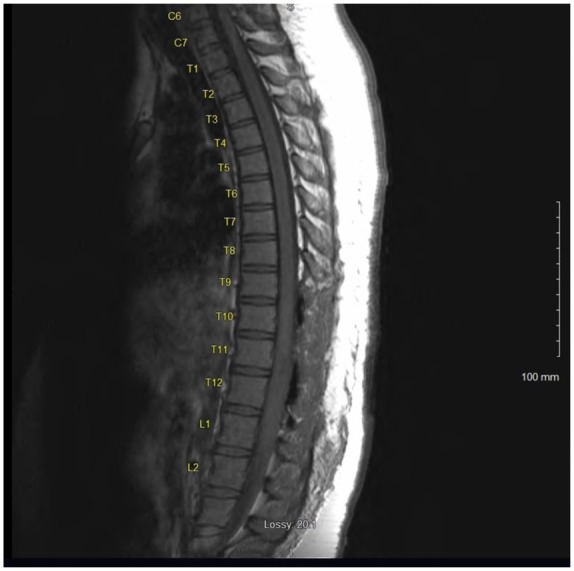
Postoperative MRI of the thoracic spine without contrast, postoperative day 1 of T9-T12 and L1 laminectomy with debulking of intramedullary spinal cord GBM.
